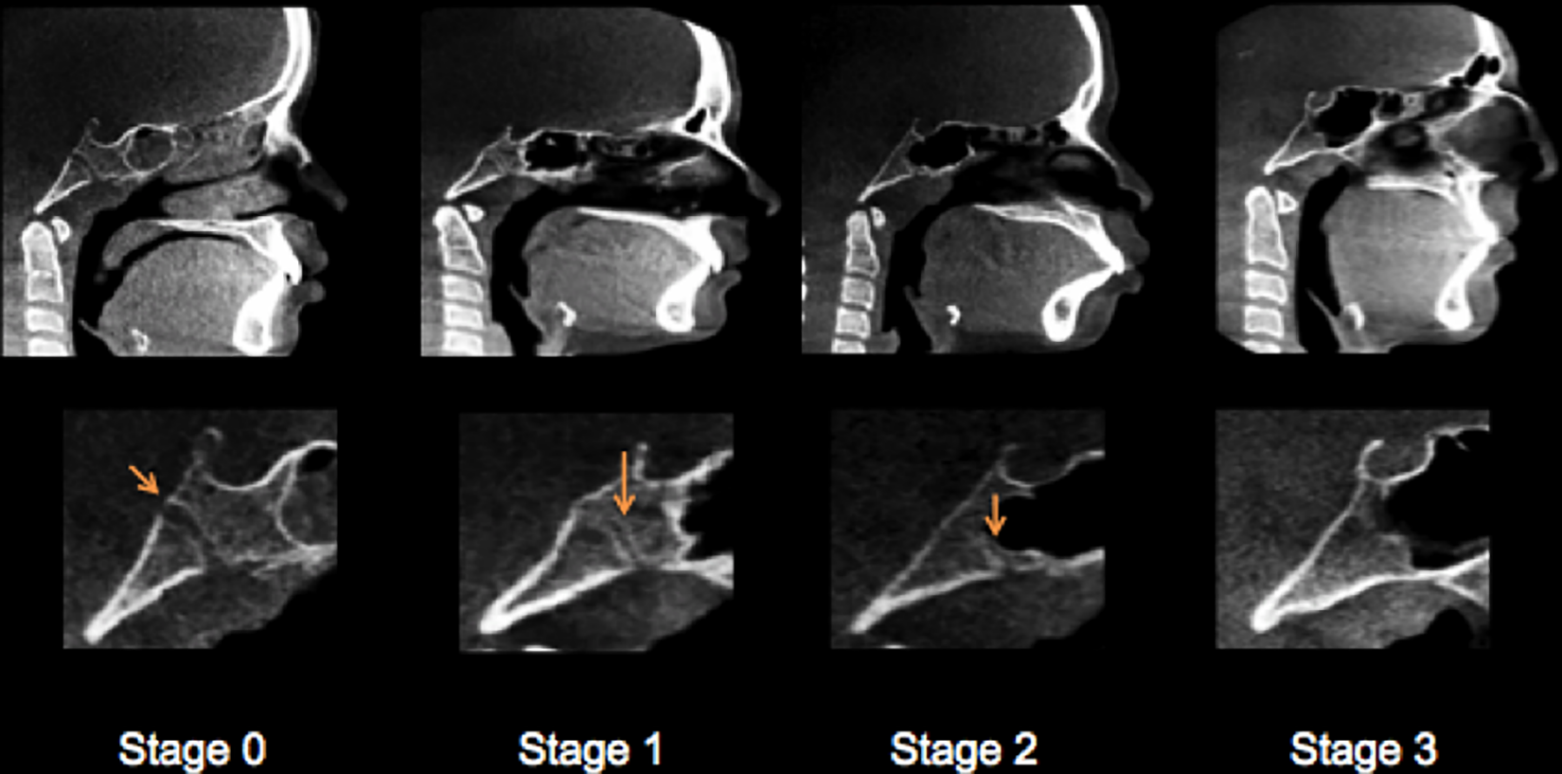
Mid-sagittal CBCT images demonstrating the spheno-occipital synchondrosis closure Stages. Stage 0, unfused. Stage 1, fusing endocranially. Stage 2, fusing ectocranially. Stage 3, complete fusion.
